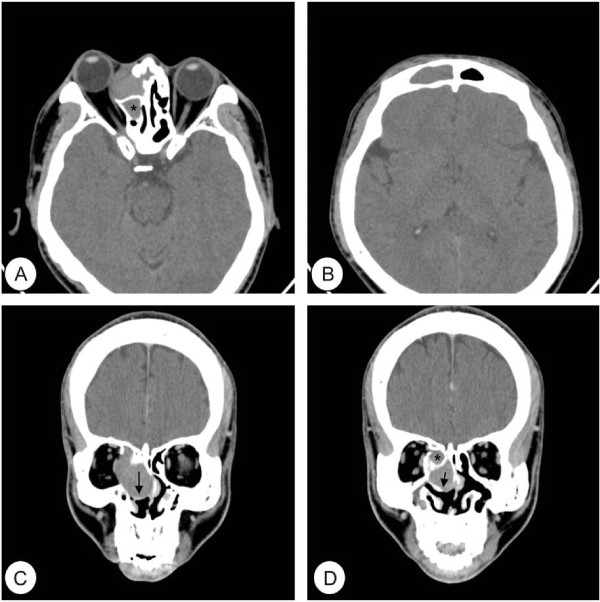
Preoperative computed tomography (CT) images of the orbit. Axial (A, B) and coronal (C, D) CT images show a well demarcated mass surrounded by a bony perimeter of the middle turbinate (arrow) at right nasal cavity. Medial orbital wall is destructed and nasal septum is deviated to the left side. The medial rectus muscle and orbit is displaced laterally. Total opacification is noted in right ethmoid sinus (asterisk) and frontal sinus.
